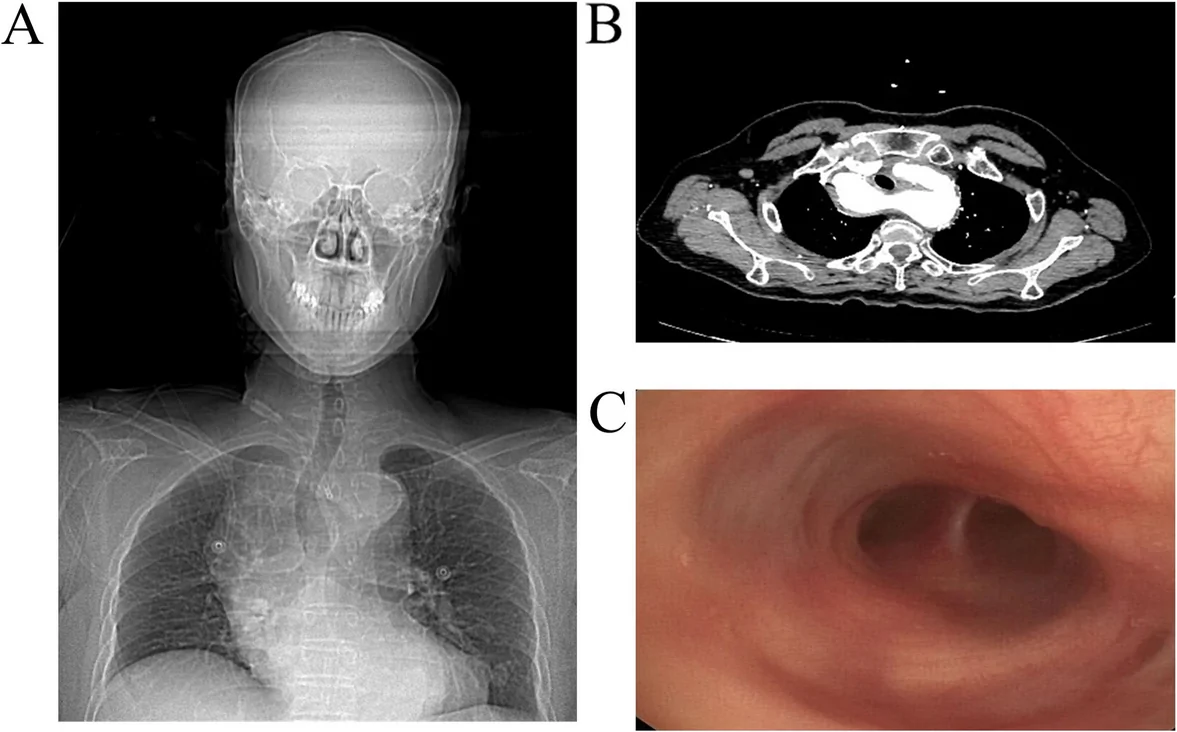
A–C Preoperative chest CT and postoperative bronchoscopy show no obvious tracheal stenosis or compression
